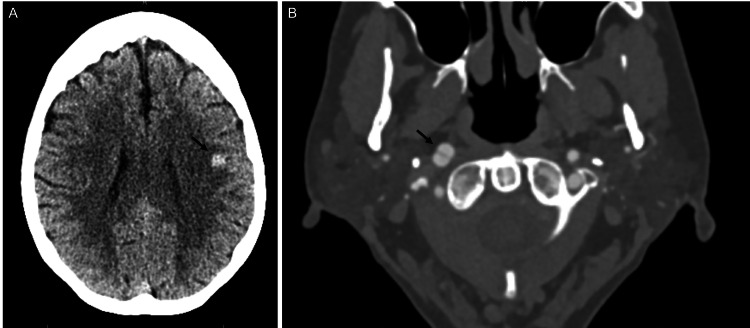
Initial CT imaging (A) Axial CT brain without contrast demonstrating left frontoparietal intraparenchymal hemorrhage; (B) Axial CT angiogram of the head and neck with contrast demonstrating dissecting pseudoaneurysm of the right internal carotid artery. Arrows are used where appropriate to indicate pathology.
